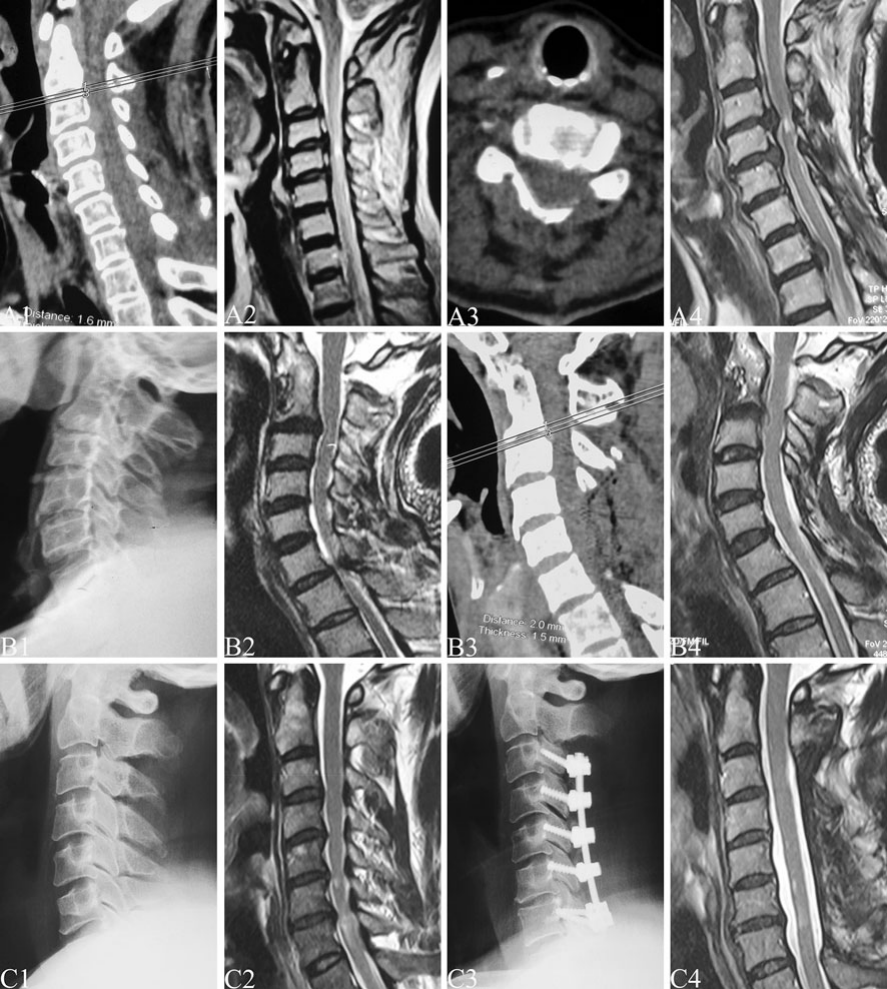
Preoperative and postoperative X-ray, CT and MRI of multilevel cervical spondylotic myelopathy treated by different posterior operations. a A 67-year-old female patient underwent C4 to C7 laminoplasty, whose preoperative JOA score was 8. In the final follow-up of 9.5 years, the loss of CI was 2.4 %, final follow-up JOA score was 14 and NDI score was 23. b A 56-year-old male patient underwent C4 to C6 laminectomy, whose preoperative JOA score was 7. In the final follow-up of 7.5 years, the loss of CI was 3.7 %, final follow-up JOA score was 13 and NDI score was 27. c A 61-year-old male patient underwent C3 to C7 laminectomy with lateral mass screw fixation, whose preoperative JOA score was 8. In the final follow-up of 8 years, the loss of CI was 0.4 %, final follow-up JOA score was 16 and NDI score was 2
